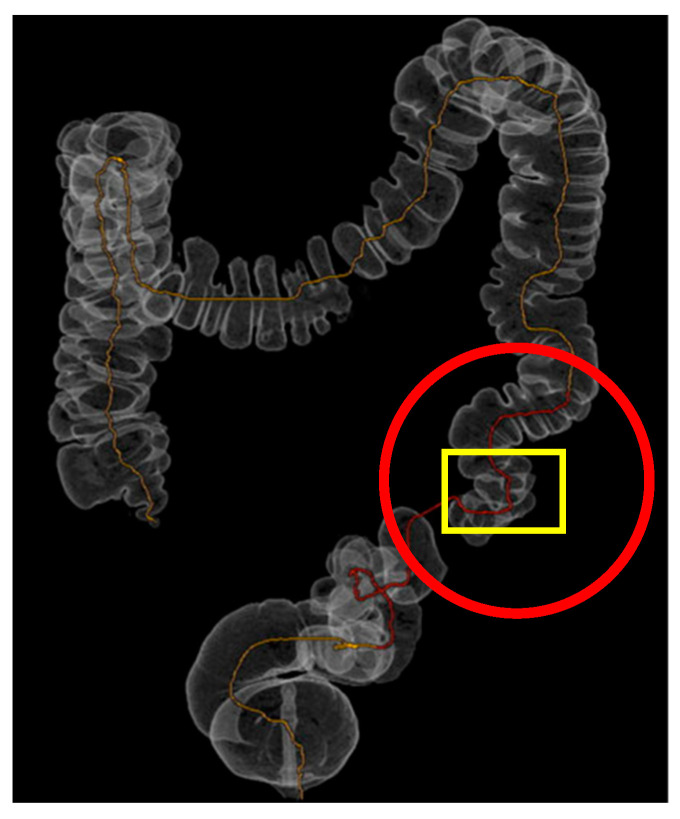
Three-dimensional rendering of CT-colonoscopy: Three-dimensional rendering of CT-colonoscopy (The diverticula are highlighted by red circles, while the stenotic segment is indicated by the yellow box).
